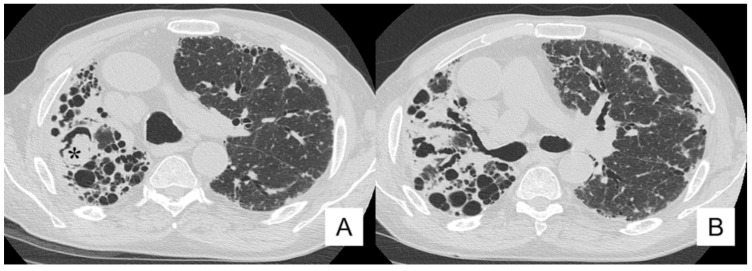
Same patient as previous figures (Figure 12 and Figure 13), HRCT acquired 2 years after the onset of IPF disease, due to clinical worsening (fever and weight loss). In the right upper lobe (A,B), we have now identified a cystic space containing dense material (black asterisk in A), resembling the monod sign. Based on this morphological appearance, the suspicion of mycotic colonization was formulated by the radiologists; after the sputum collection, a final diagnosis of pulmonary aspergilloma was made.
